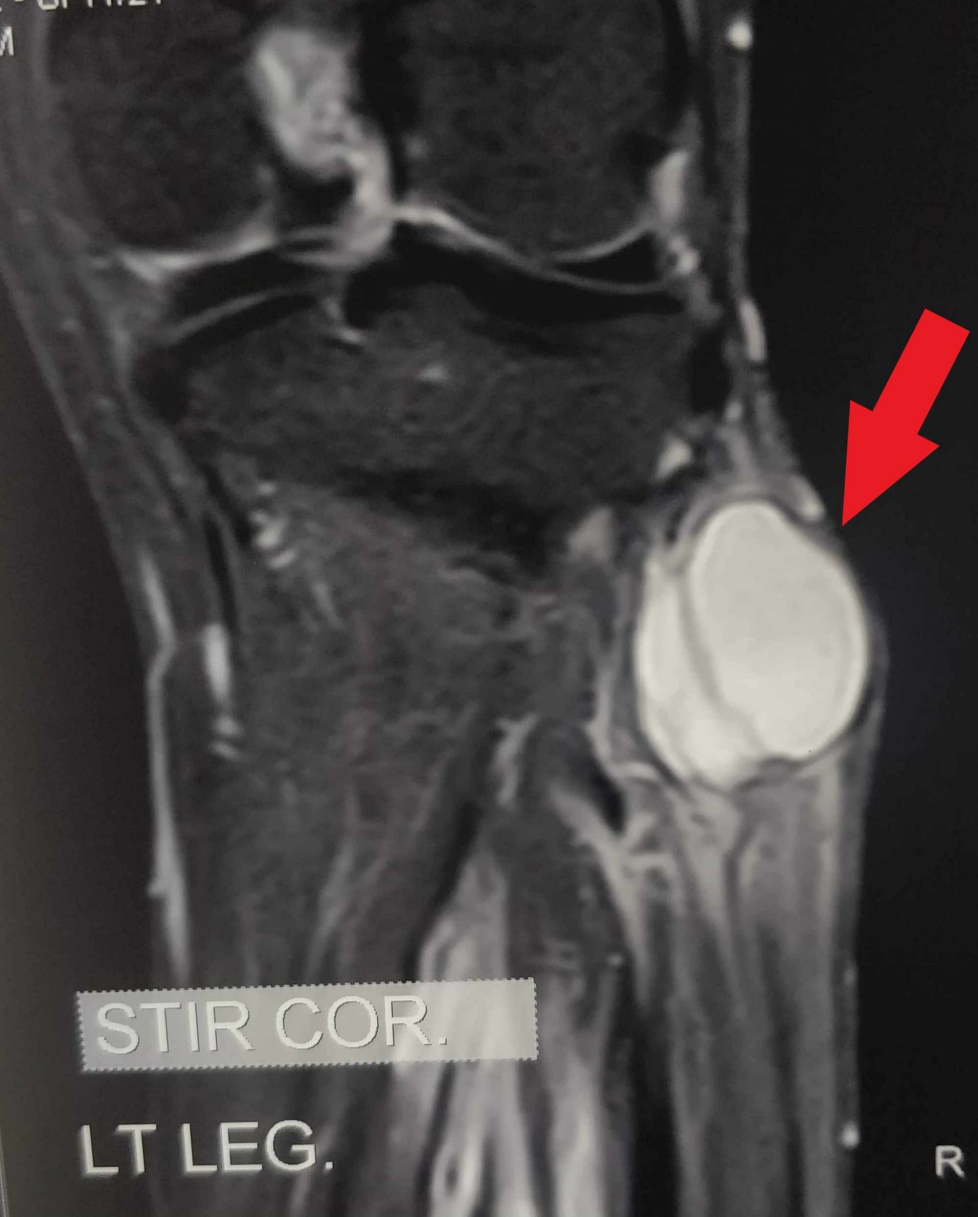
Coronal cut of magnetic resonance imaging showing ganglion cyst (pointed by the red arrow)
